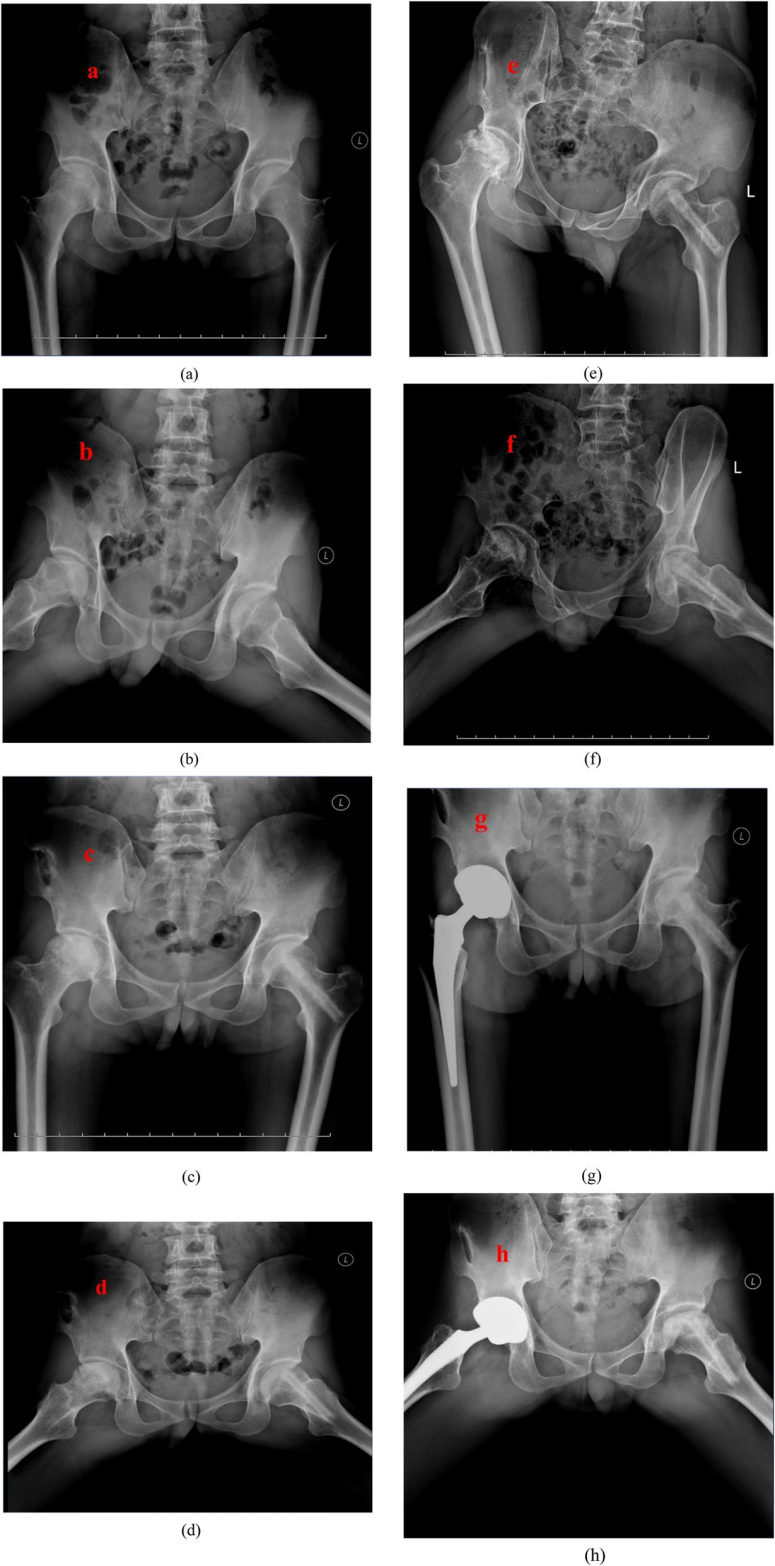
a, b The 38-year-old man was diagnosed with bilateral osteonecrosis of the femoral head due to alcohol overtake. c, d Postoperative 1-year X-rays showed both femoral head shape was maintained and the cystic area was reduced or eliminated, which indicates the necrotic repair response was good. e, f Postoperative 6-year X-rays revealed right femoral head collapsed and secondary osteoarthritis occurred, while the left hip was completely healed. g, h The patient had a right hip total hip arthroplasty conversion, while the left hip was maintained
